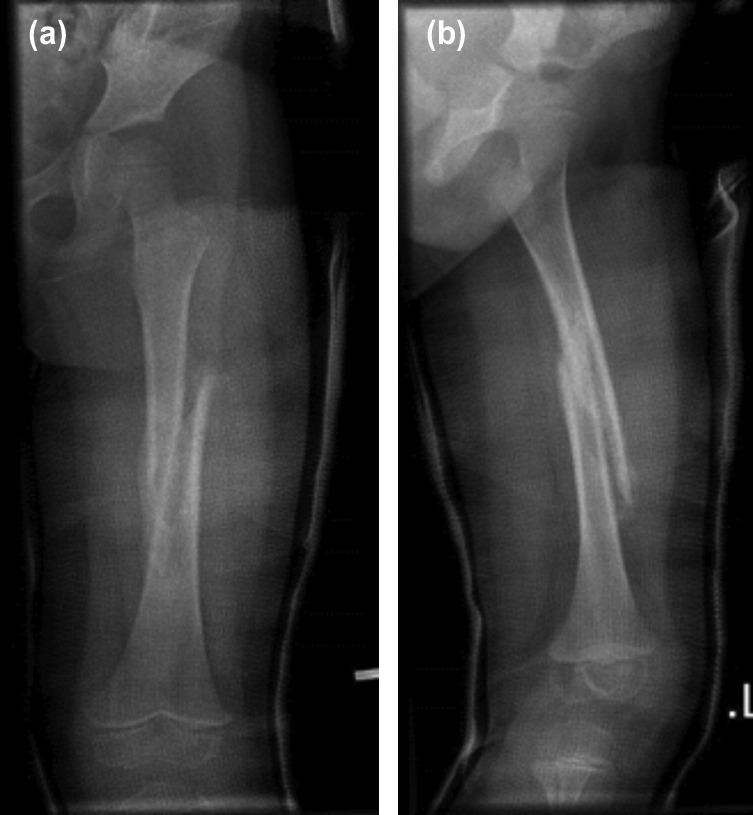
(a,b) Radiographs showing loosening of the initial cast around the thigh especially proximally after four weeks for patient A.
